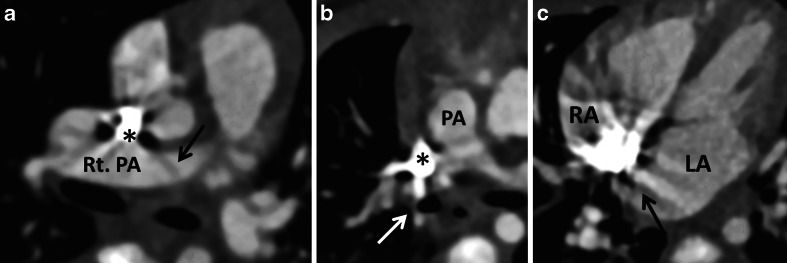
Example images of beam hardening artifacts due to a dense contrast medium in the superior vena cava (*). a Contrast-enhanced CT transverse images of a 26-day-old girl. A beam hardening artifact (Grade 2, *arrow*) may have affected the evaluation of the right pulmonary artery (Rt. PA). b Contrast-enhanced CT images of a 29-day-old boy. Beam hardening (Grade 3, *arrow*) made it hard to evaluate the pulmonary artery (PA). c Contrast-enhanced CT transverse images of a 3-month-old boy. A beam hardening artifact (Grade 3, *arrow*) disturbed the depiction of the atrium septum. *LA* left atrium, *RA* right atrium
